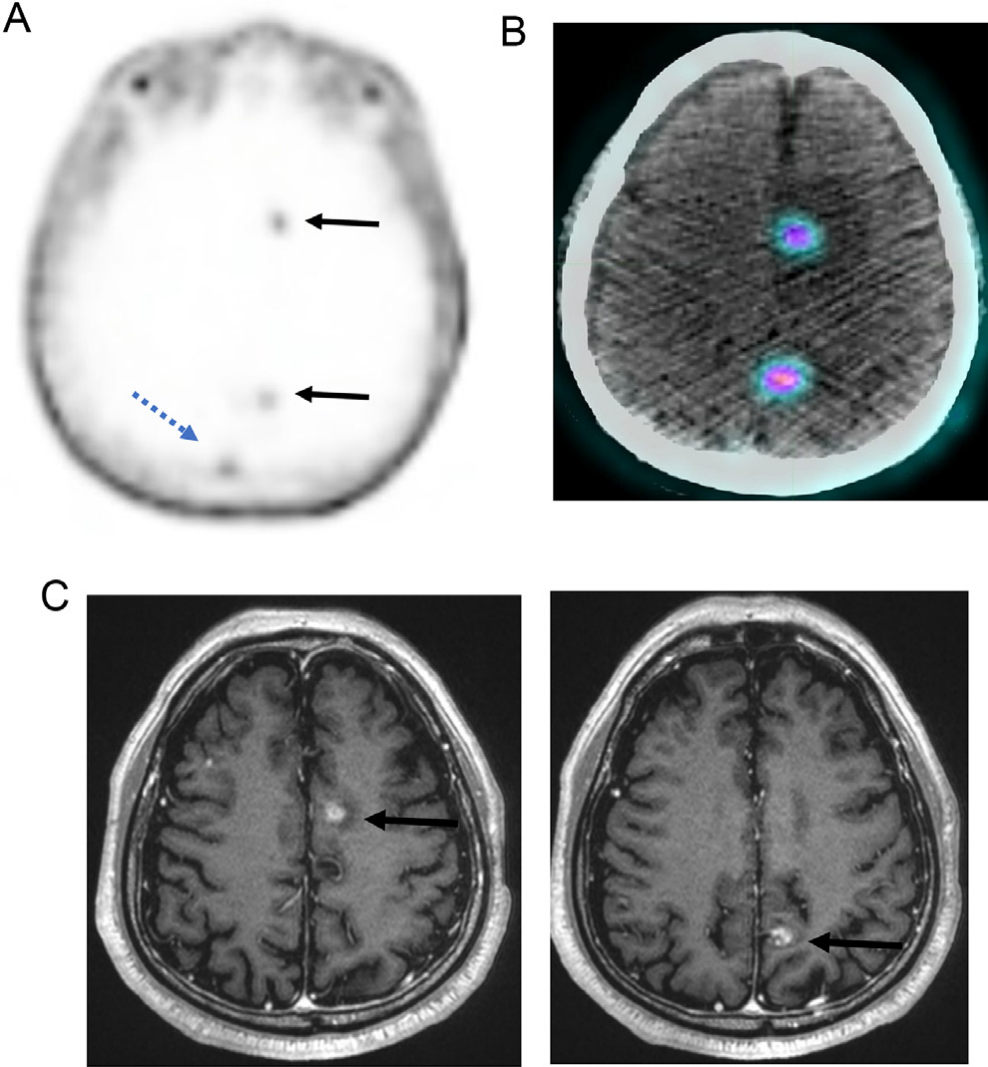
(A) PSMA PET post radiation shows regression of lesions with SUV 1.2 to 1.4 (solid arrows) similar to blood pool SUV 1.4 (dotted arrow) over venous sagittal sinus. (B) PET/CT fusion image shows lesions with low activity compared to baseline. (C) MR T1 with contrast post radiation shows dramatic reduction in lesion size.
